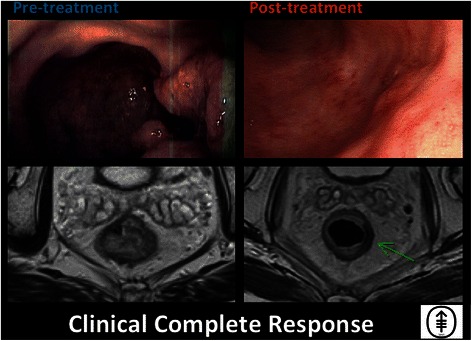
Clinical complete response. Endoscopic and T2-weighted MRI images, both pre- and post-treatment, are shown for a patient who has achieved a clinical complete response. Images displayed were taken from endoscopic and MRI views of an 85 year-old man who underwent capecitabine CRT followed by consolidation chemotherapy with CapeOx and was determined to achieve cCR both clinically and radiologically. In the post-treatment T2-weighted MRI image shown, the green arrow points to the prior site of the tumor
